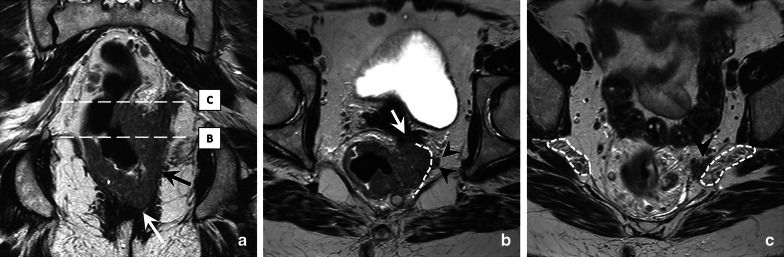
Coronal T2-weighted image (a) and axial T2-weighted cross sections at two different levels (b, c) of a 48 year old female patient with a cT4b rectal tumour based on invasion of the left external anal sphincter (white arrow in a; = skeletal muscle invasion), invasion of the pelvic floor (black arrow in a; = skeletal muscle invasion), invasion of the obturator compartment (arrowheads in b; = invasion of compartment outside the mesorectum), and invasion of the vagina (white arrow in b; = organ invasion). There is also a close relation to the sacral nerve plexus (white dotted lines) on the left side (arrowhead in c)
